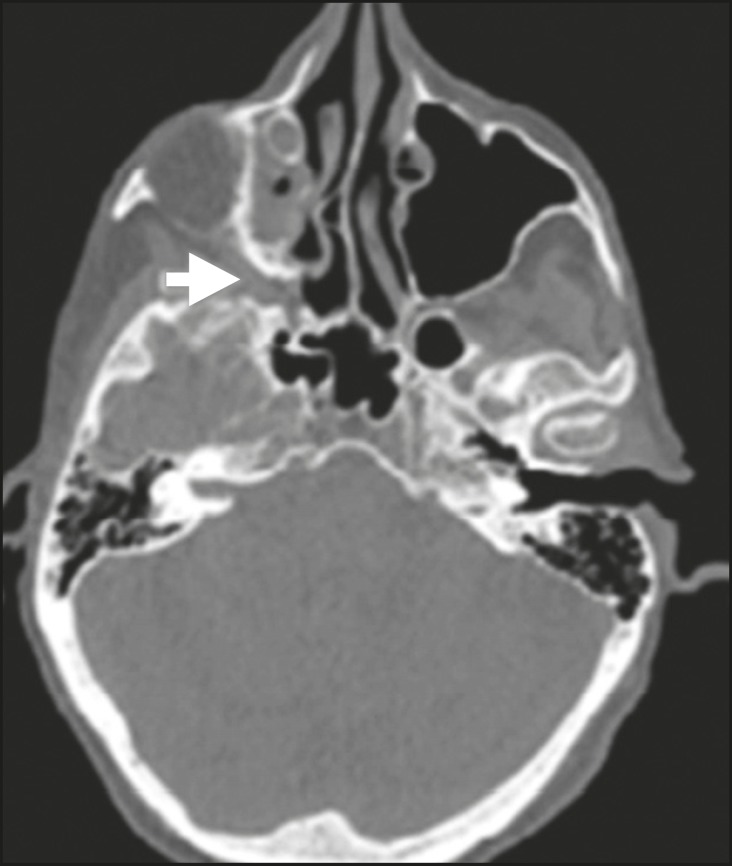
Contrast-enhanced CT scan of a 90- year-old female with squamous cell
